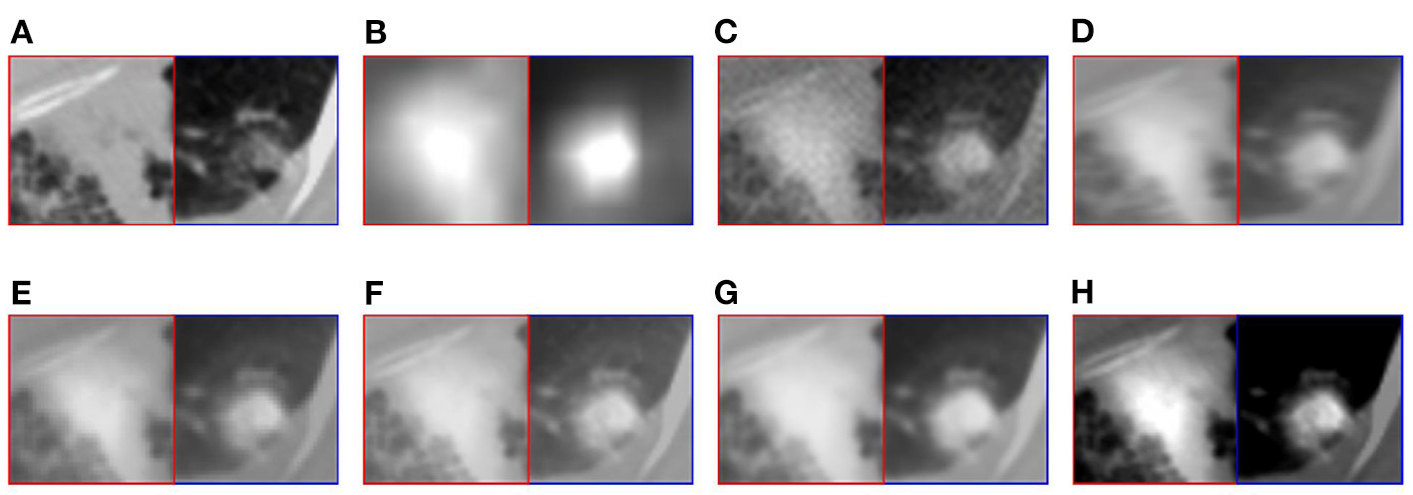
The detail of fusion results of patient A. (A) CT; (B) PET; (C) AD; (D) GF; (E) PAPCNN; (F) MCFNET; (G) IFCNN; (H) OURS.
